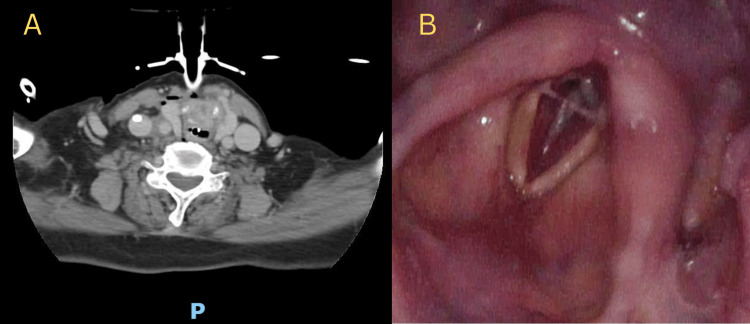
Axial view of contrasted neck CT scan (A) and bronchoscopy image at vocal cord level (B) showing severe subglottic stenosis with posterior glottis gap of about 2 mm
